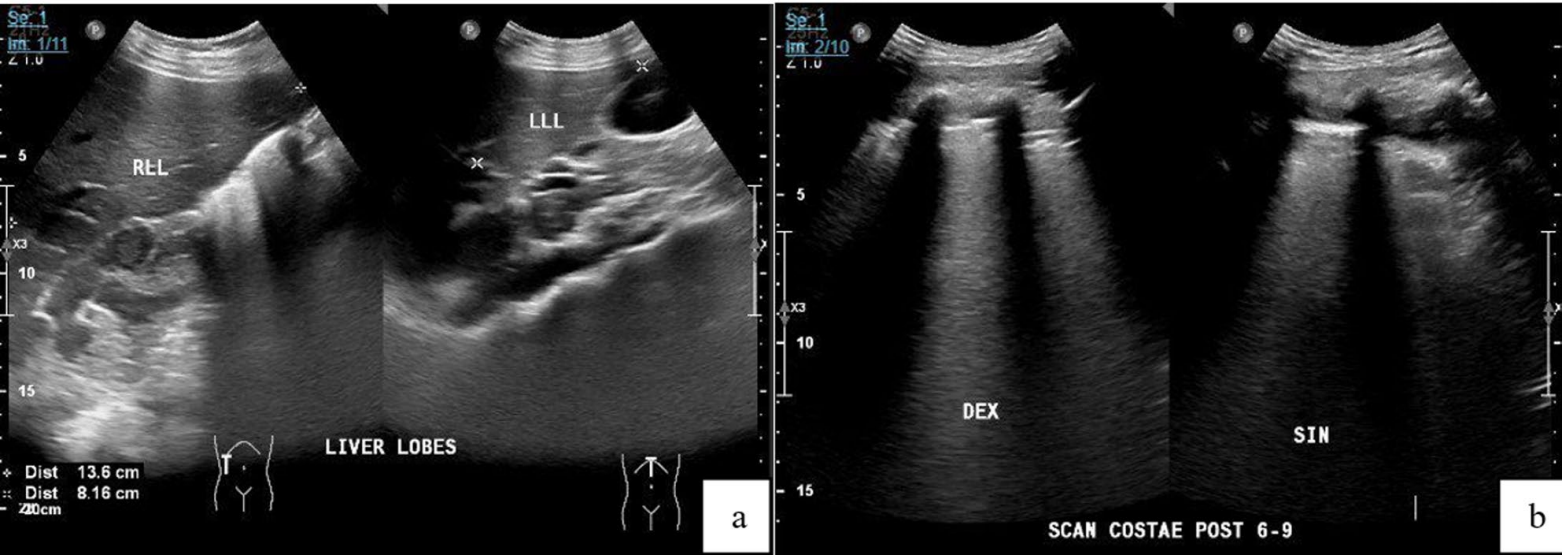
Ultrasonography of the patient (a) Abdomen and (b) Thorax
